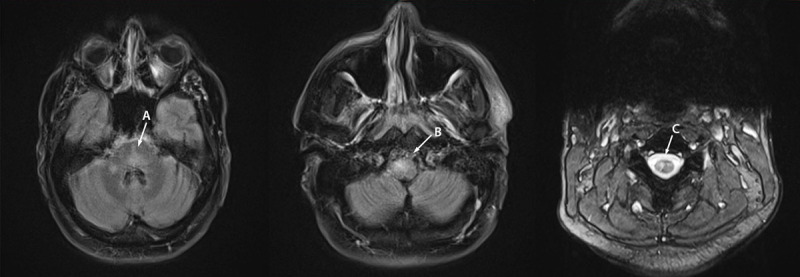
Magnetic resonance imaging of the brain and cervical spine of patient B with monkeypox-associated encephalomyelitis showing abnormal T2/fluid attenuated inversion recovery signal in the pons and cerebellum (A), medulla (B), and gray matter of the cervical spinal cord (C) — District of Columbia, July–August 2022 Photos/Matthew J. Copeland.
